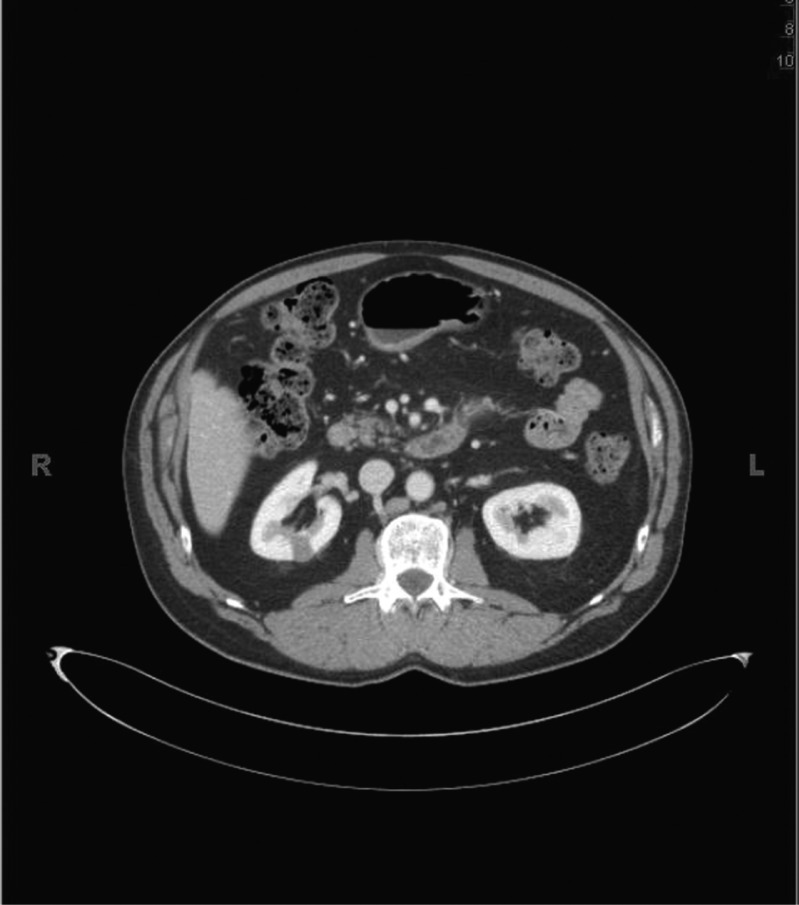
Abdominal Computed Tomography scan image of the patient with HPRC: The abdomen CT scan with contrast of the patient showing a well defined homogeneous hypodense mass of 1.3×1.9 cm in the right kidney.
